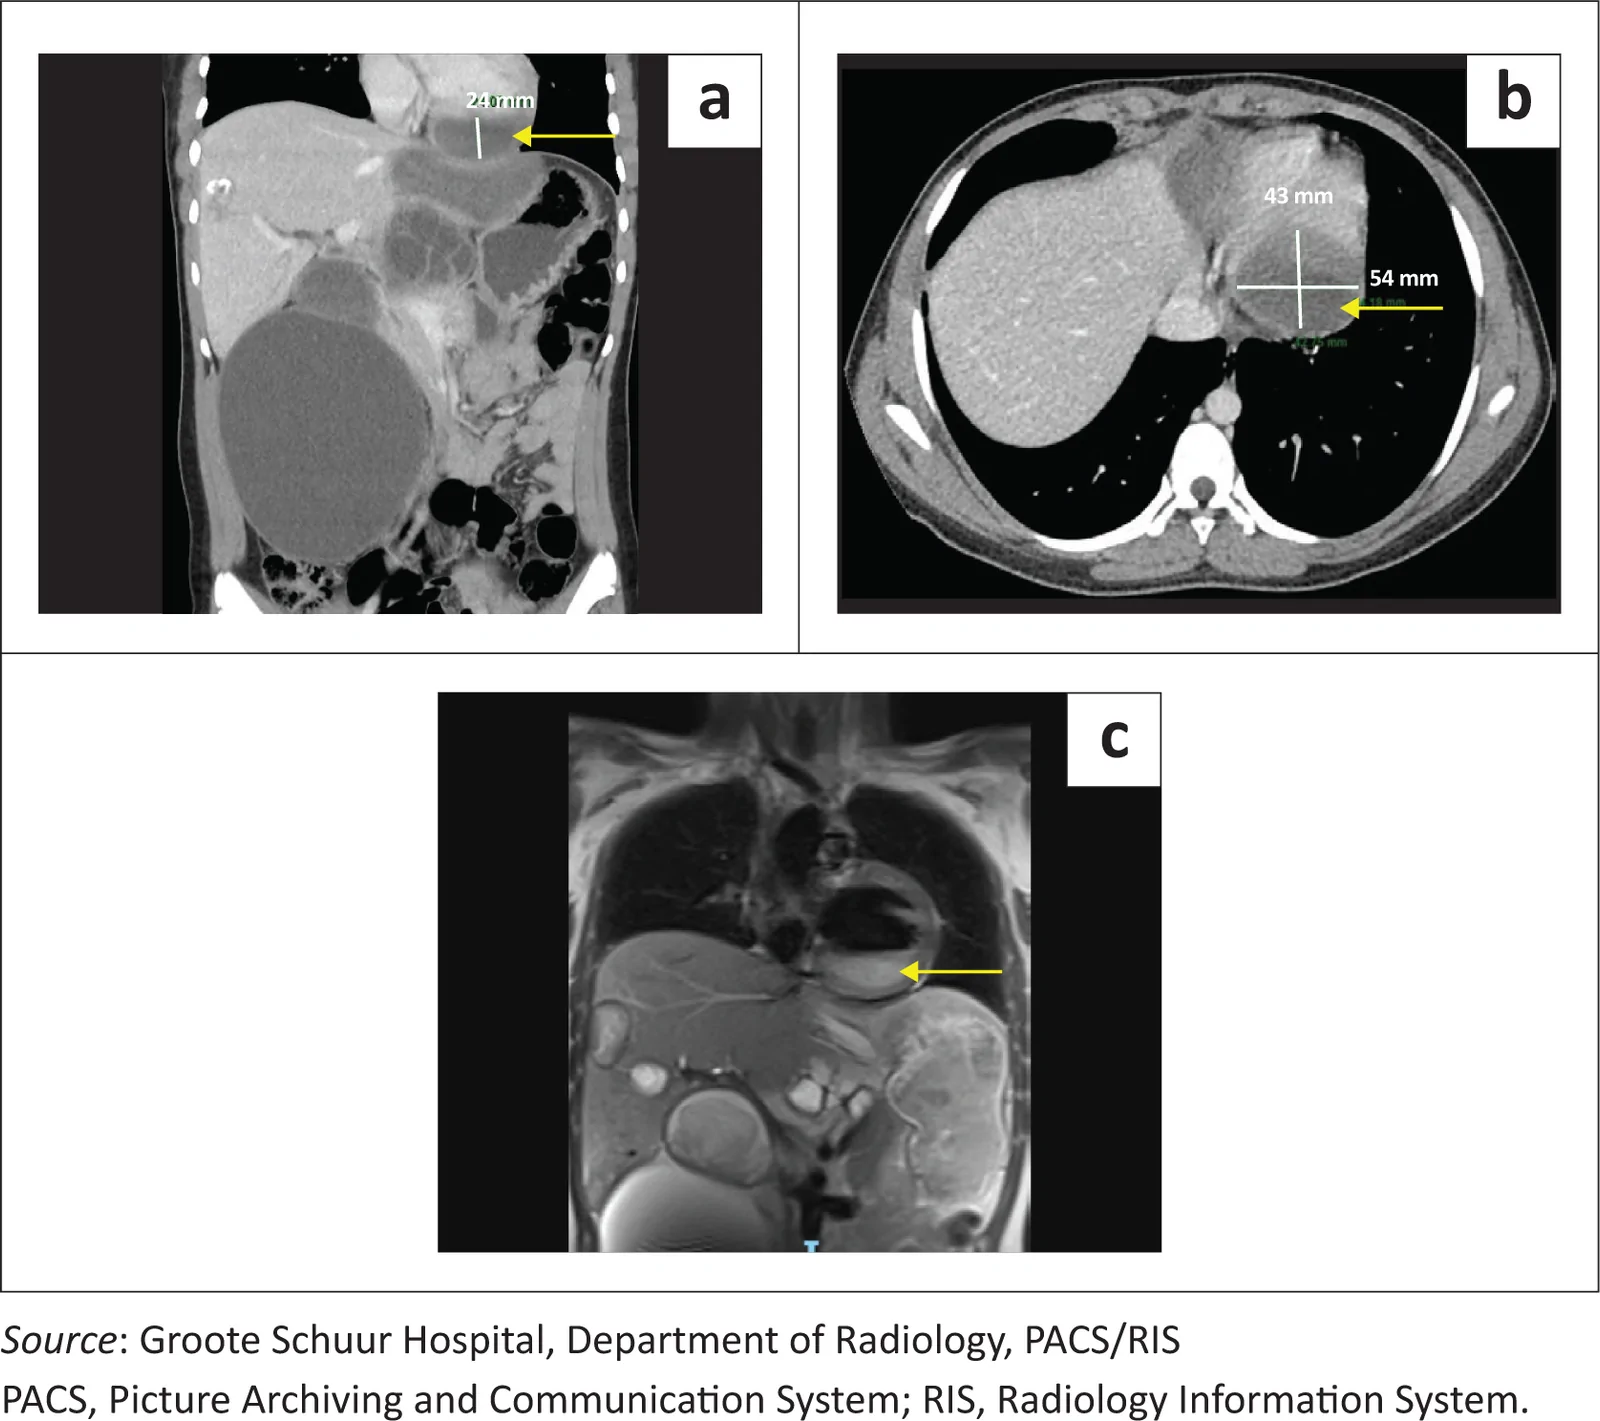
Contrast-enhanced coronal (a) and axial (b) CT and coronal T2 MRI (c) of a 17-year-old HIV-negative female patient with hepatic and extrahepatic cysts and, notably, a myocardial cyst, measuring 54 mm × 43 mm × 24 mm (anteroposterior, transverse, craniocaudal) (arrows).
